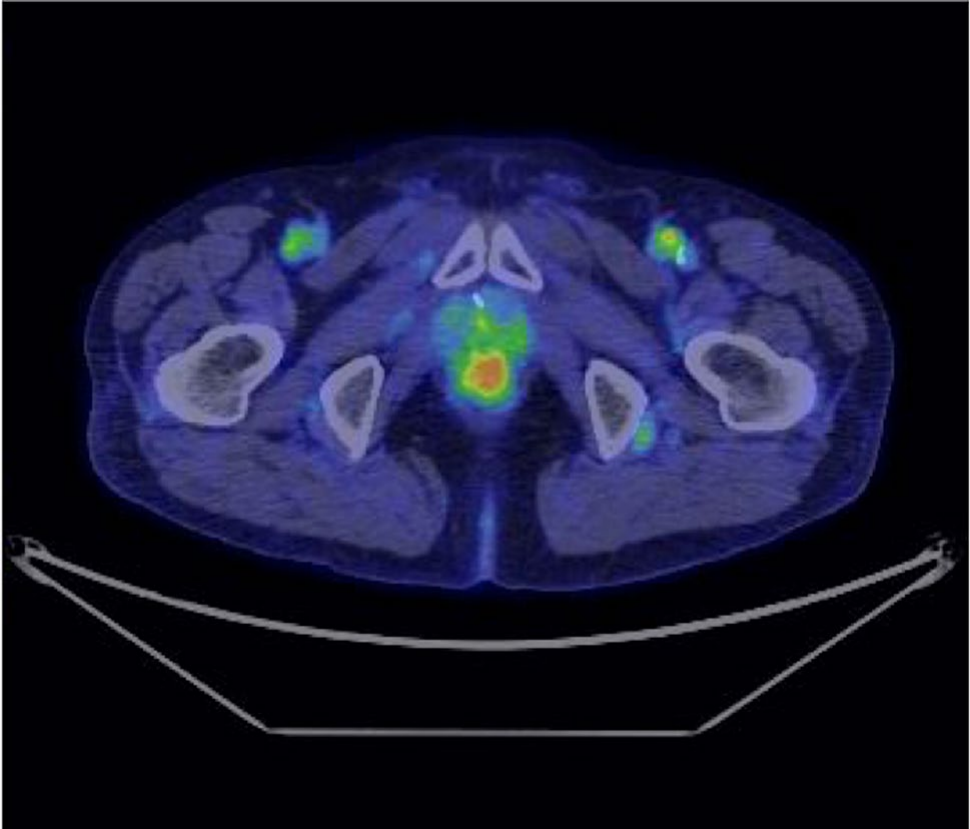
PET–CT detected FDG accumulation with a maximum standardized uptake value (SUV max) of 2.22 in the rectum
